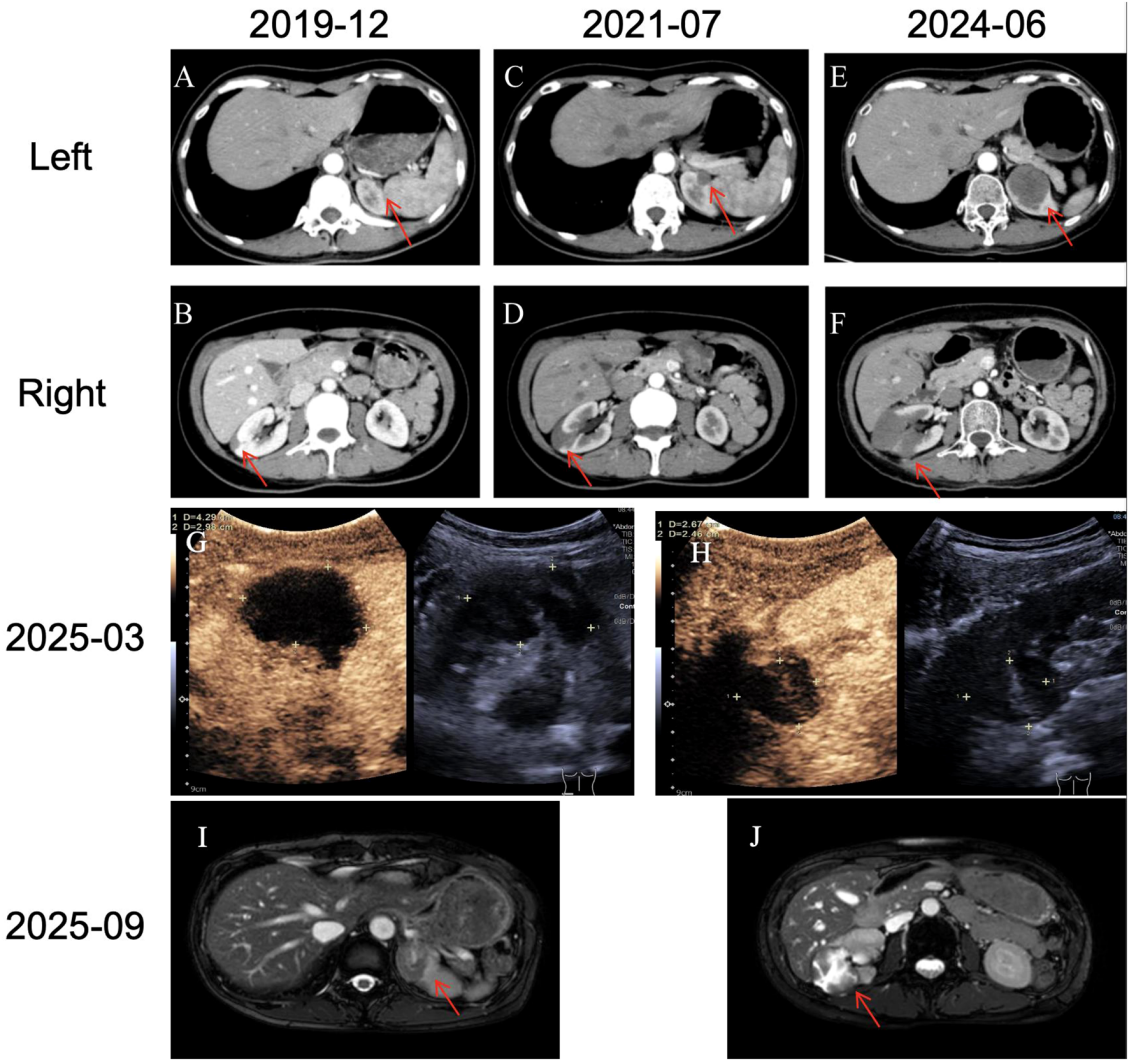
Follow-up images of renal metastases. (A-F) The changes in the location and size of bilateral renal masses on imaging. Contrast-enhanced ultrasound of both kidneys: (G) 27×25mm on the left side; (H) Two masses on the right side, the larger one being 27×25mm. No enhancement was observed on both sides. (I, J) MR suggested changes in bilateral renal masses after treatment, with no obvious activity.
